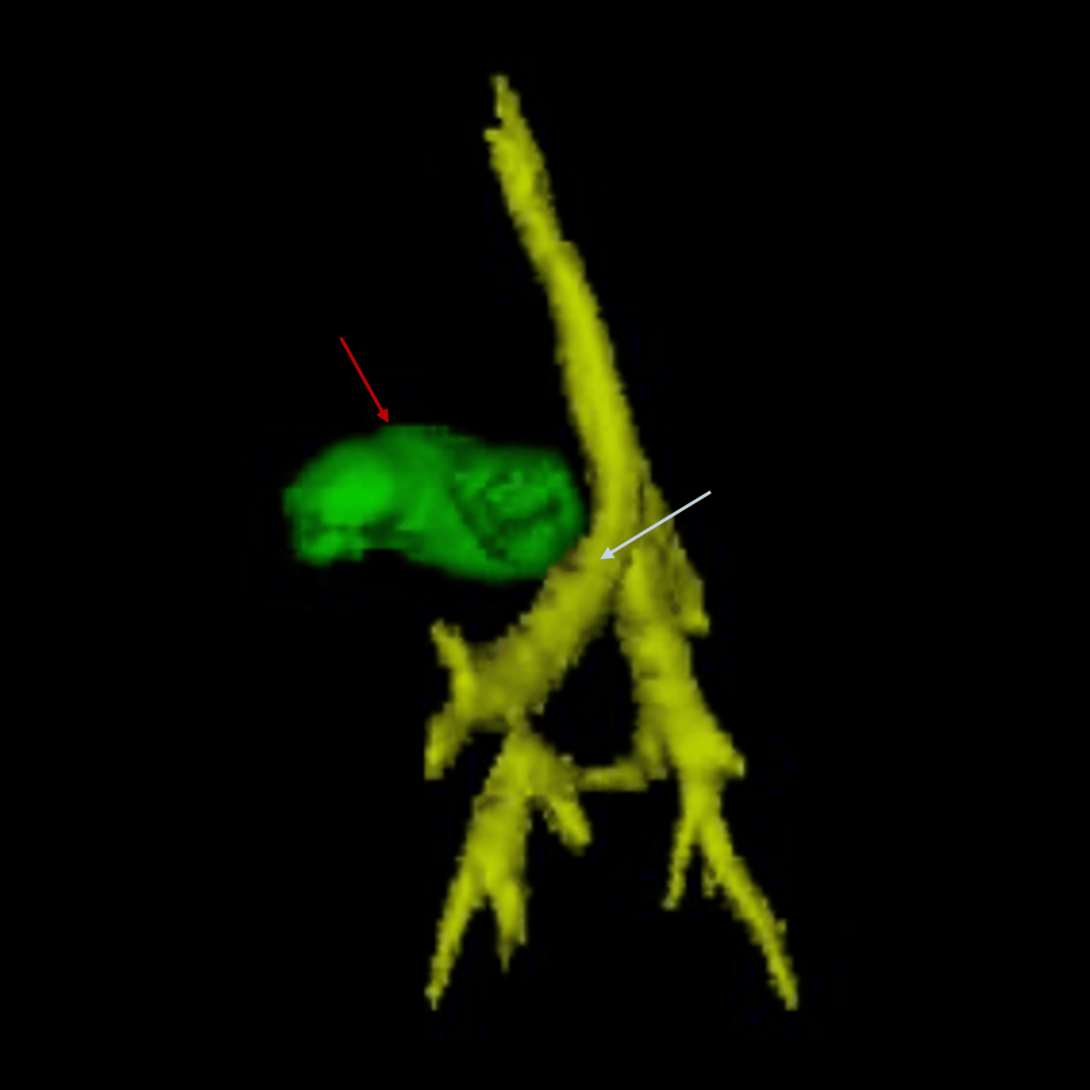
Three-dimensional reconstruction of a computed tomography image showing compression of the left main bronchus (white arrow) by an enlarged, tortuous patent ductus arteriosus (red arrow), resulting in airway obstruction.
